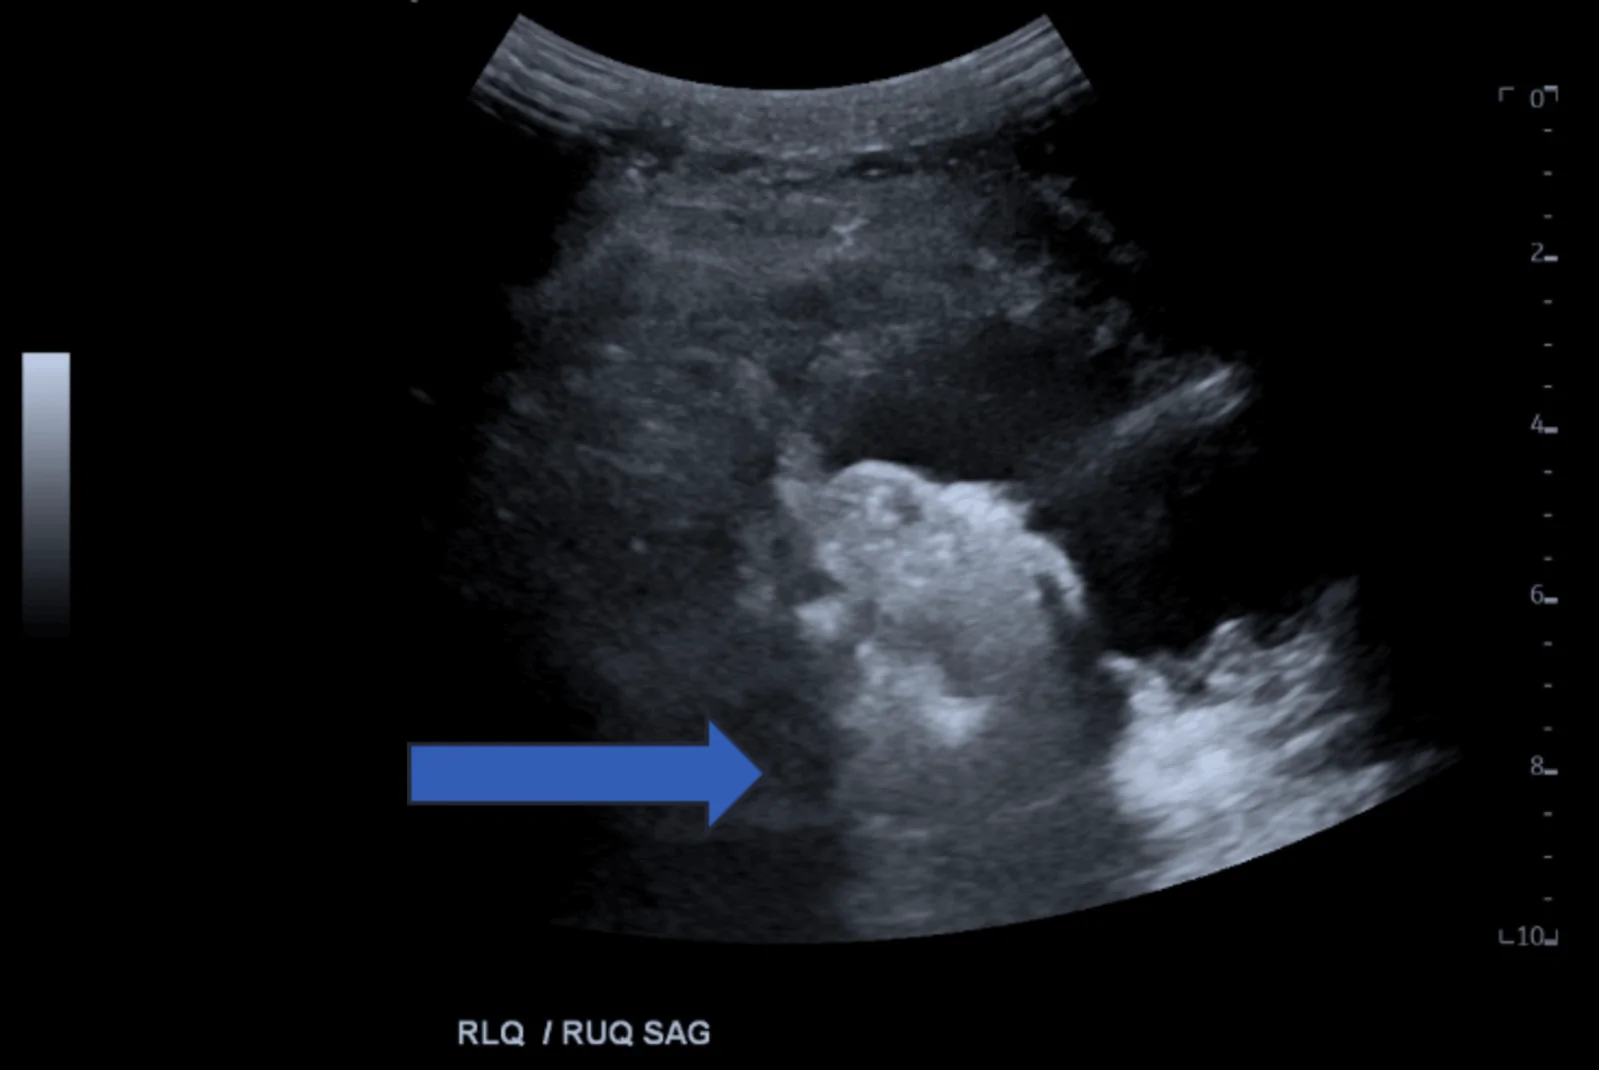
Abdominal ultrasound demonstrating the intraabdominal mass in the right lower quadrant identified during emergency department evaluation RUQ: right upper quadrant; RLQ: right lower quadrant; SAG: sagittal
